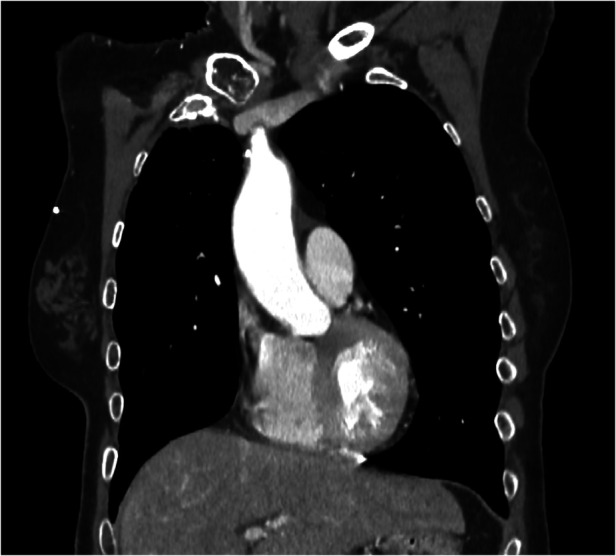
Coronal CT-scan plane showing the resolution of the 8 mm thrombus in the ascending aorta.
